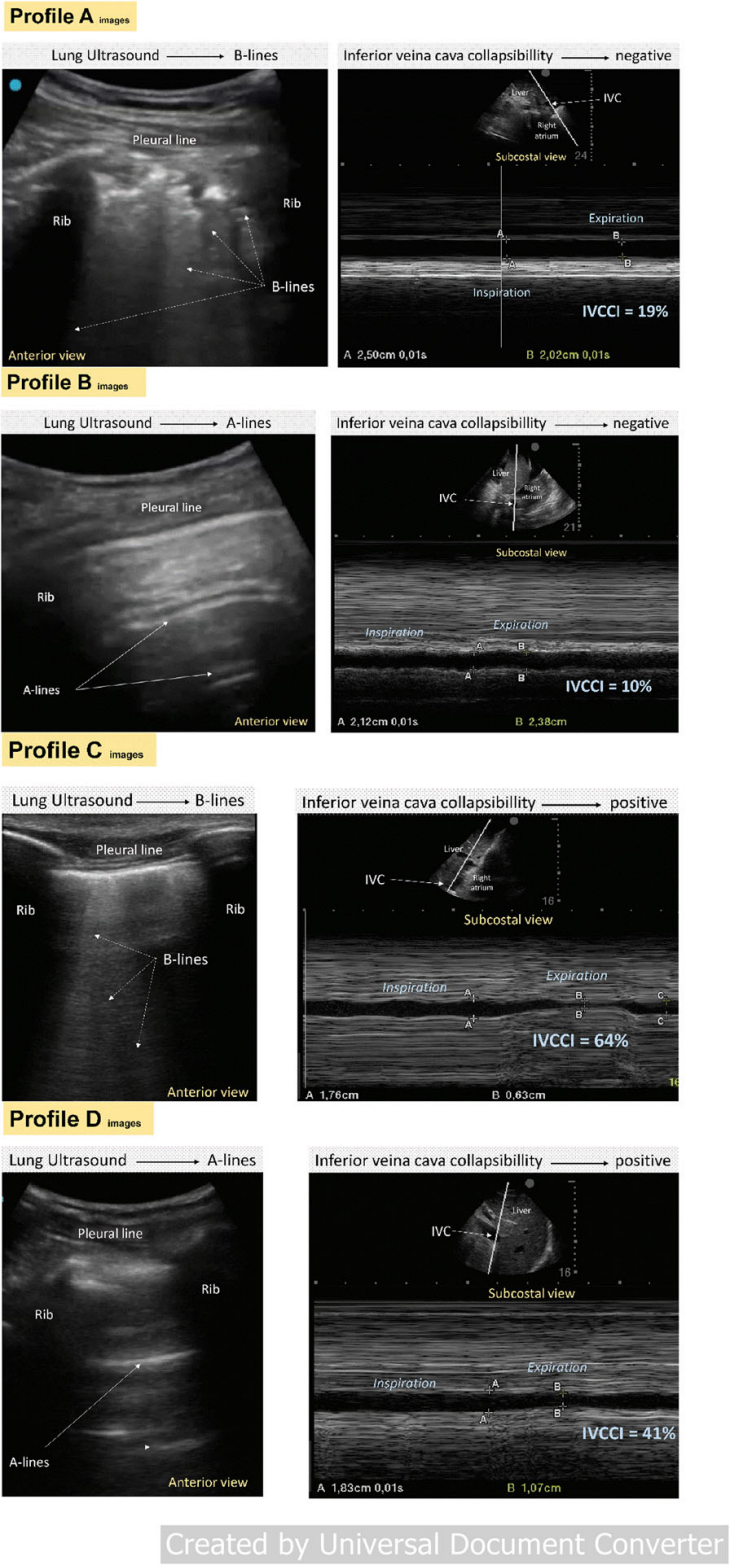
Vena cava and lung ultrasound profiles. (Profile A) 66-year-old patient admitted to the intensive care unit for community-acquired pneumonia with acute kidney injury KDIGO 3 requiring hemodialysis. The patient had a positive fluid balance of 6.5 L and did not receive vasoactive support. In the right upper anterior thoracic region, multiple coalescent B lines issued from justapleural consolidations typical of bronchopneumonia are visible. As shown in the corresponding video file (Additional files 1, 2, 3, and 4), lung sliding is nearly abolished caused by inflammation/infection of pleural layers. The vena cava appears well filled and does not show any significant collapsibility with respiratory movements. (Profile B) 28-year-old patient admitted to the intensive care unit for a urinary tract infection, and acute kidney injury KDIGO 3 requiring dialysis was diagnosed. The patient had a positive fluid balance of 2.5 L, and he was on vasoactive support. In the right upper anterior thoracic region, predominant A lines are visible. As shown in the corresponding video file (Additional files 1, 2, 3, and 4), lung sliding is normal. The vena cava appears filled and shows not significant collapsibility with respiratory movements. (Profile C) 53-year-old patient admitted to the intensive care unit for an alcoholic acute pancreatitis. AKI (KDIGO) 2 was diagnosed, and intermittent dialysis was initiated. The patient had a positive fluid balance of 8.5 L, and he was on vasoactive support. In the right upper anterior thoracic region, white lines from the pleural line to the bottom (B–lines–comets) are visible. As shown in the corresponding video file (Additional files 1, 2, 3 and 4), lung sliding is normal. The vena cava appears collapsed with respiratory movements. (Profile D) 73-year-old patient admitted to the intensive care unit for an acute mesenteric ischemia treated by an extended right hemicolectomy. The patient developed AKI KDIGO 3, and intermittent dialysis was started. The patient had a positive fluid balance of 5.5 L and did not receive vasoactive support. In the right upper anterior thoracic region, horizontal lines (A lines) are visible. As shown in the corresponding video file (Additional files 1, 2, 3, and 4), lung sliding is normal. The vena cava appears collapsed with respiratory movements
